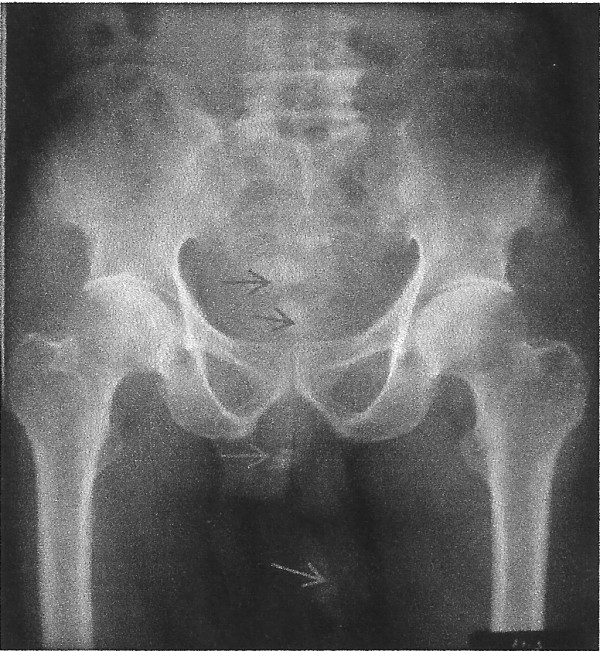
Plain radiograph showing diverticulum, bladder neck, and urethral stones (arrow).
